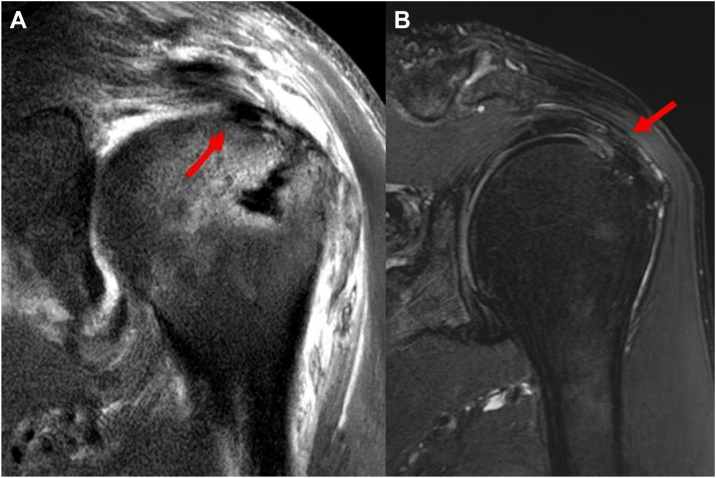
Postoperative MRI outcomes of the same patient showing successful revision repair. (A) A postoperative coronal T2-weighted image demonstrates the augmented biceps tendon (*red arrow*) well-seated beneath the supraspinatus tendon, effectively filling the articular-sided defect. (B) A postoperative sagittal oblique view shows the restored continuity and structural integrity of the rotator cuff with the biceps augmentation (*red arrow*). *MRI*, magnetic resonance imaging.
